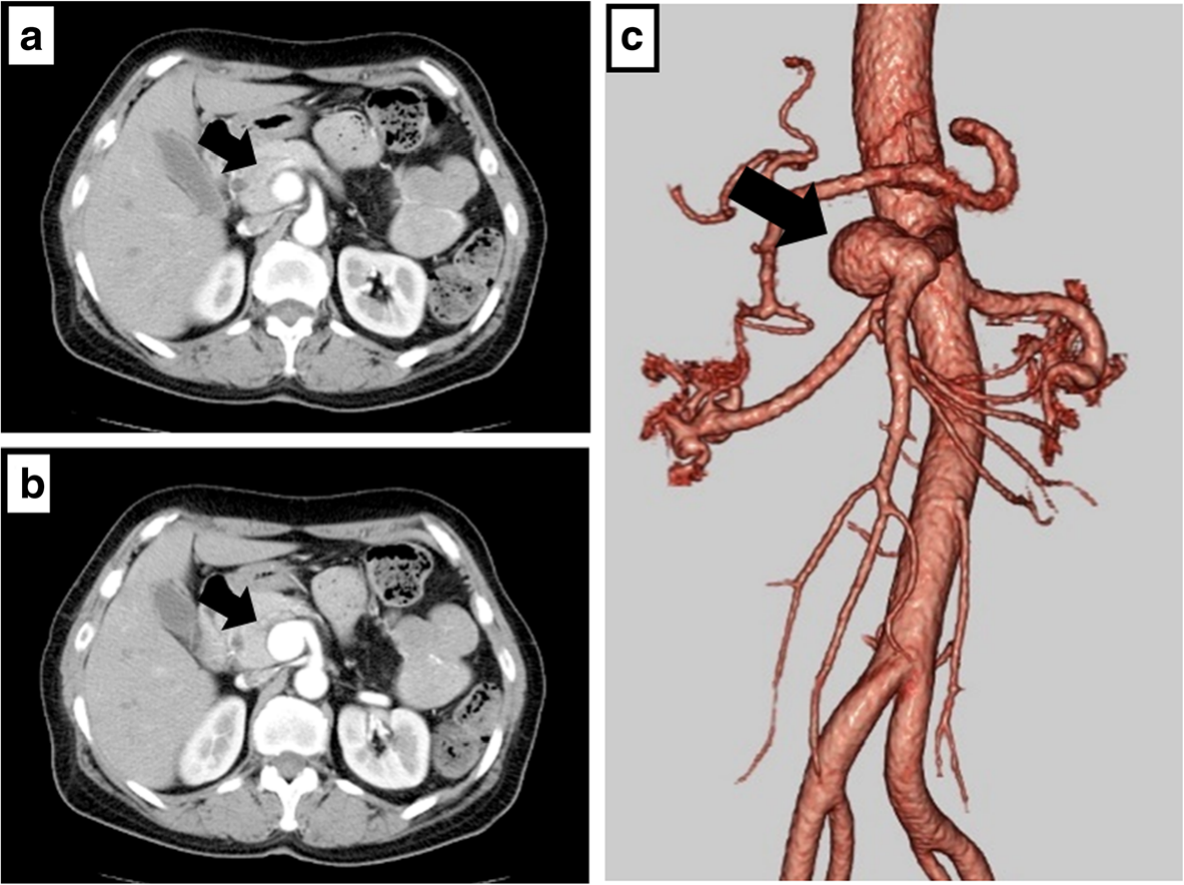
Axial contrast-enhanced computed tomography (a, b) and 3-dimensional image reconstruction (c) showing a 2.4-cm saccular aneurysm of the superior mesenteric artery (arrow)
